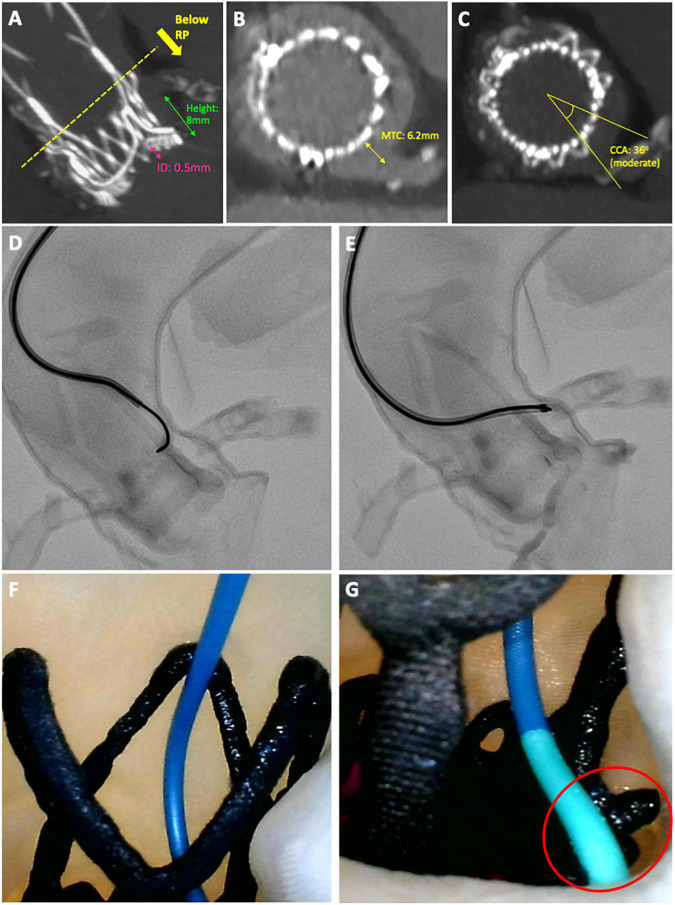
Case example of a challenging diagnostic cannulation. An 83-year old male underwent ACURATE neo implantation to treat a 14-year old degenerated Perimount23 surgical bioprosthesis. Post-procedural CT showed (A) a high implantation with low-lying coronary arteries, (B) a narrow gap between valve frame and aortic wall and (C) moderate overlap between the commissural posts and coronary arteries, all implying challenging cannulation. (D,E) Semi-selective cannulation of the LCA was achieved using an Amplatz Left 2 guiding catheter with 0.014 wire-assistance, after 17 min of fluoroscopy time and four attempts. (F,G) Camera placed internally demonstrating cannulation technique of approaching the ostium from above and resting the distal tip of the guiding catheter on the upper crown of the ACURATE neo adjacent to the LCA.
